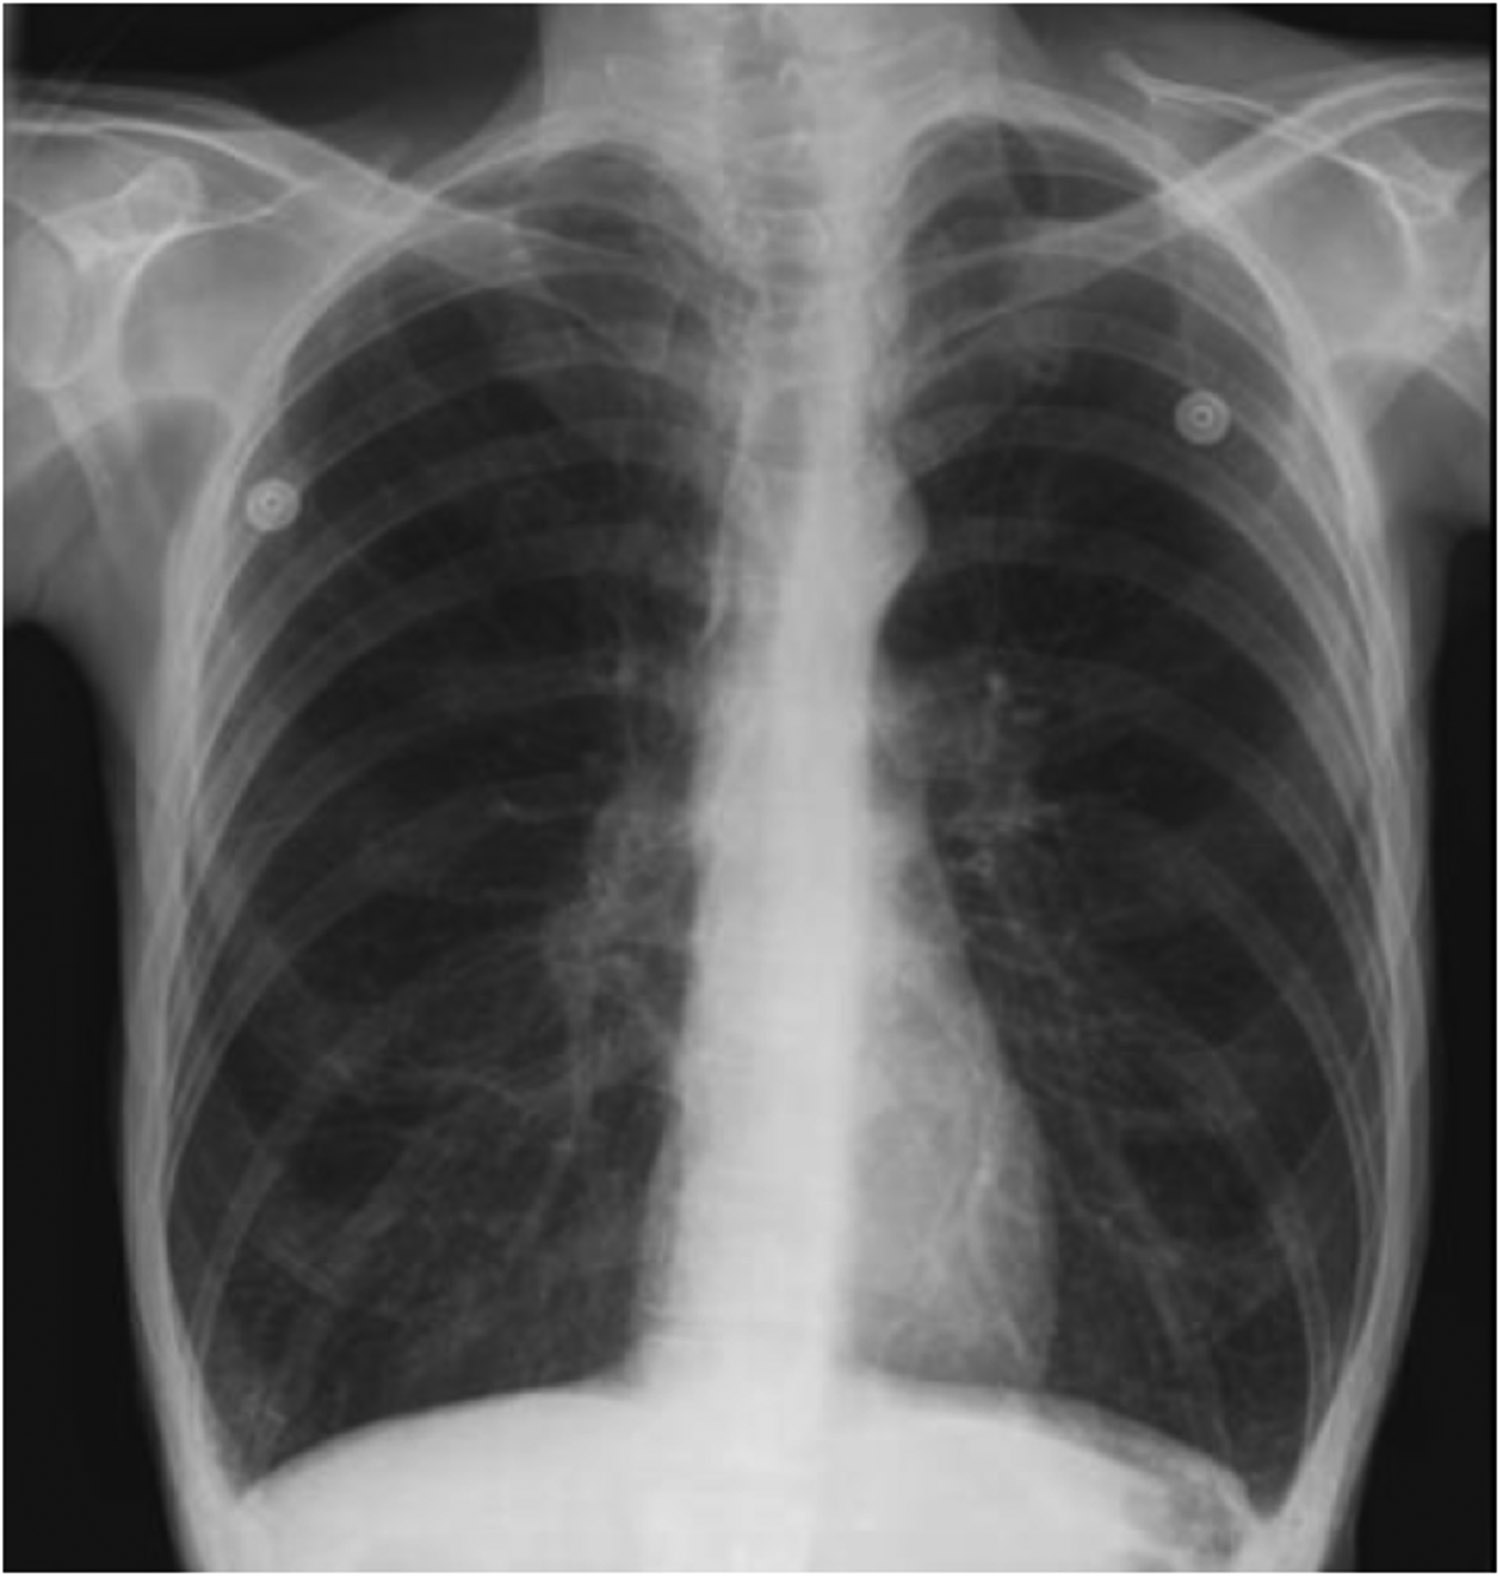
Representative chest X-ray of bronchospasm. During acute bronchospasm there is hyperinflation of the lungs with compression of the heart into a tubular shape and displacement of the diaphragm in a caudal direction. The increase in intrapulmonary pressure may lead to decreased venous return to the heart and a decrease in cardiac output.
